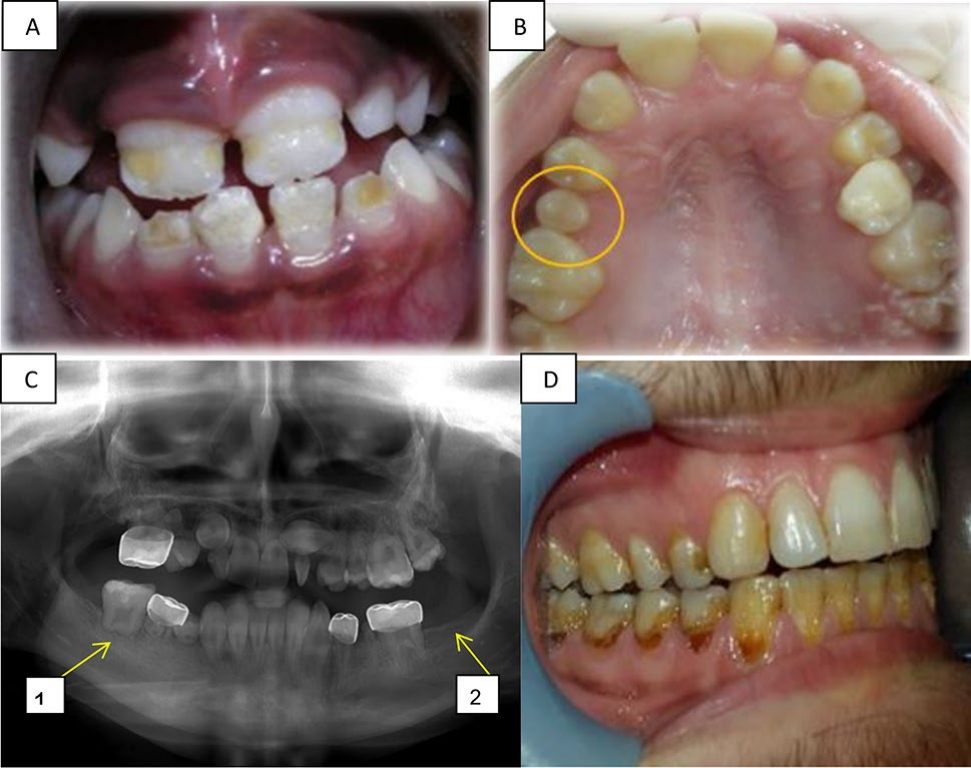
Long term dental effects. (A) Hypoplasia in the front upper and lower teeth of a girl aged 9 years, treated for ALL at age 3.5 years. (B) Microdontia showing the second upper right premolar in a girl age 12 years, treated for neuroblastoma at age 4 years. (C) A panoramic radiograph of a 12-year-old boy diagnosed with Burkitt's lymphoma at age 4 years, revealing: C_1_. Altered root development at the first lower right molar, C_2_. Hypodontia of the second lower left molar. (D) Radiation caries in a 21-year-old boy treated for neuroectodermal tumor at age 14 years.
